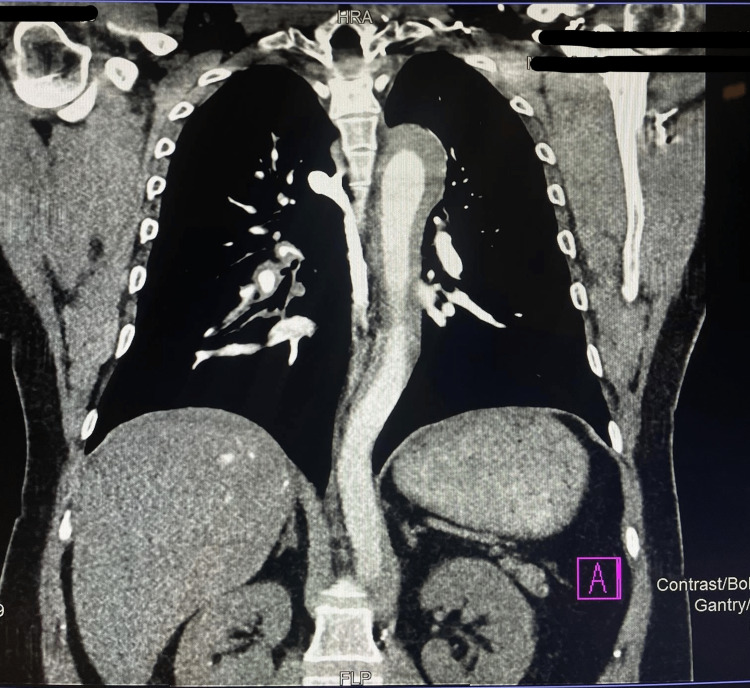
CT angiography of the chest showing intramural hematoma thrombosed on the descending thoracic aorta
